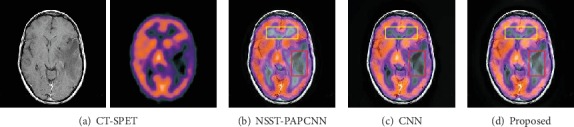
Fusion results of different methods of CT-SPET image.
